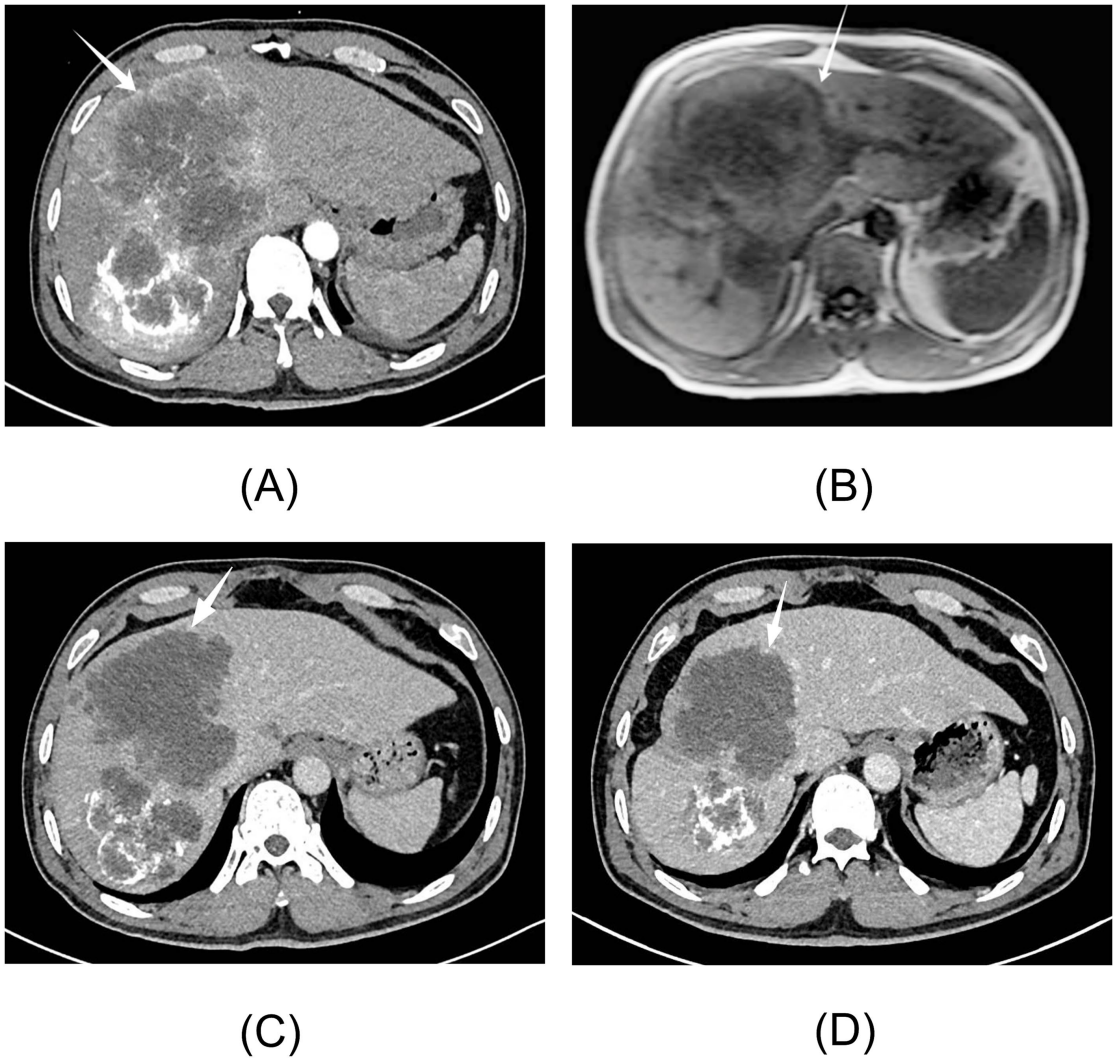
Imaging findings of Case 2. (A) Contrast-enhanced CT of the abdomen at initial diagnosis. An intrahepatic space-occupying lesion with a maximal cross-section of 18.1 × 13.1 cm was found. (B) Unenhanced and hepatocyte-specific contrast-enhanced liver MRI at initial diagnosis. An intrahepatic lesion with a maximal cross-section of 11.8 × 13.5 cm was found. (C) The tumor diameter was 10.8 × 9.6 cm after the first TACE. (D) The tumor diameter was 8.3 × 8.1 cm after the third TACE. TACE, transcatheter arterial chemoembolization.
